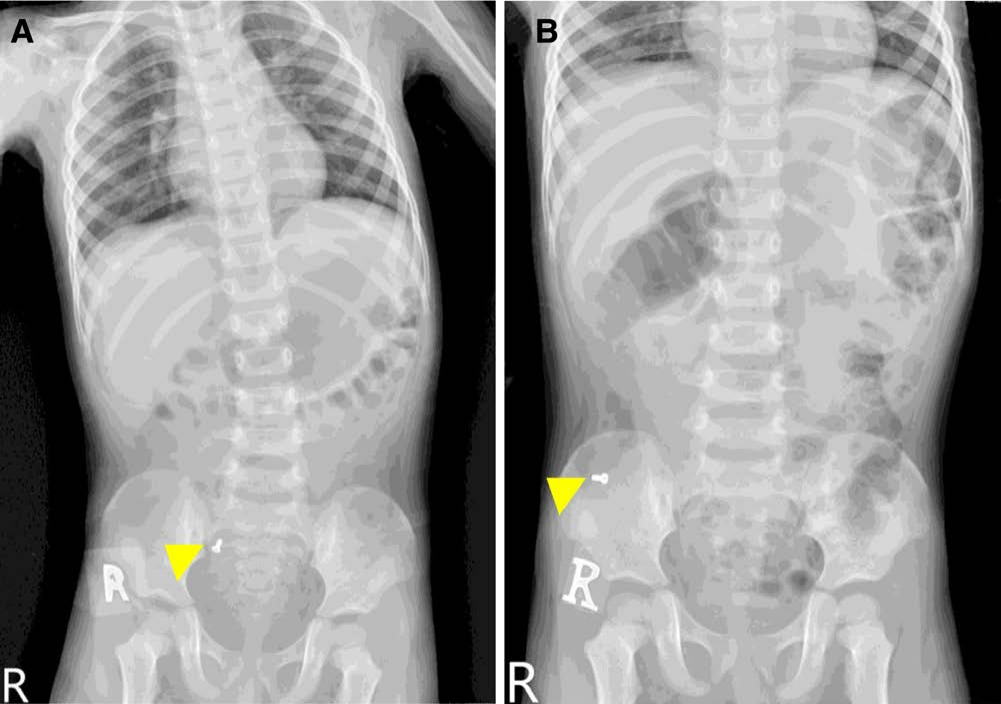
Foreign body screw (arrowhead) in right lower abdomen on (A) abdominal radiography and (B) serial abdominal radiography approximately 1 mo later.
